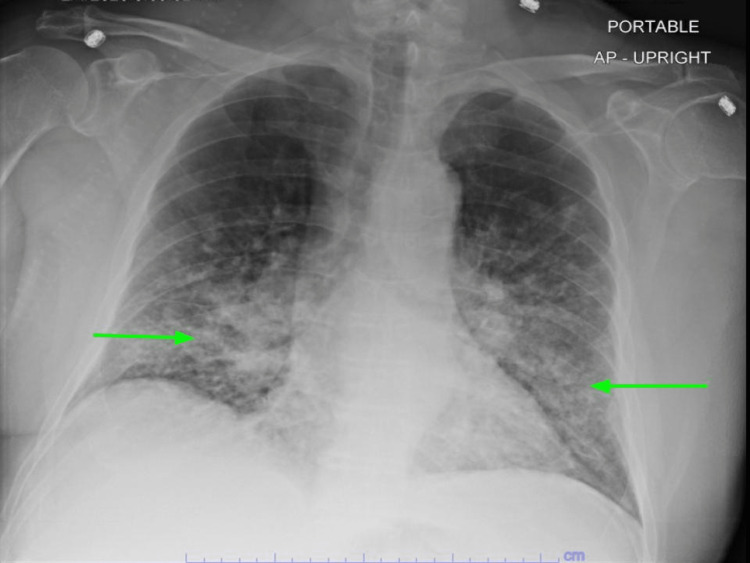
CXR showed increased patchy airspace disease throughout the mid- and lower-lung zones bilaterally (green arrows), suggesting multifocal pneumonia. CXR: Chest radiography
